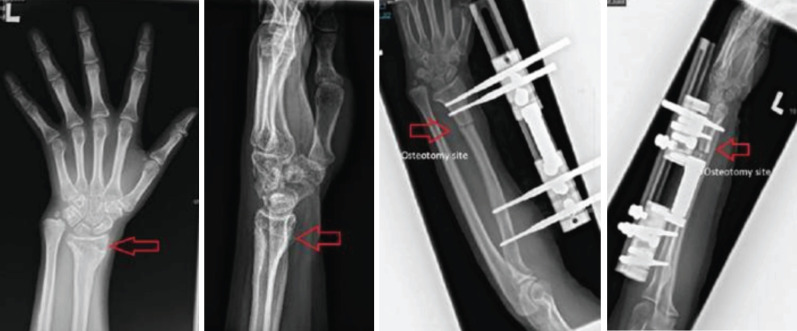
Radiograph on left – AP and lateral with left radius shortening radiograph on right -AP and lateral post osteotomy and LRS of left radius.
